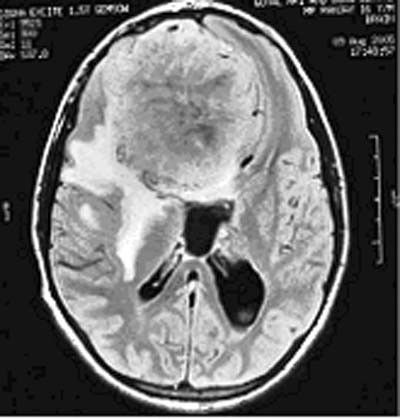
MRI scan showing a large bifrontal hyperintense mass with central necrosis.
